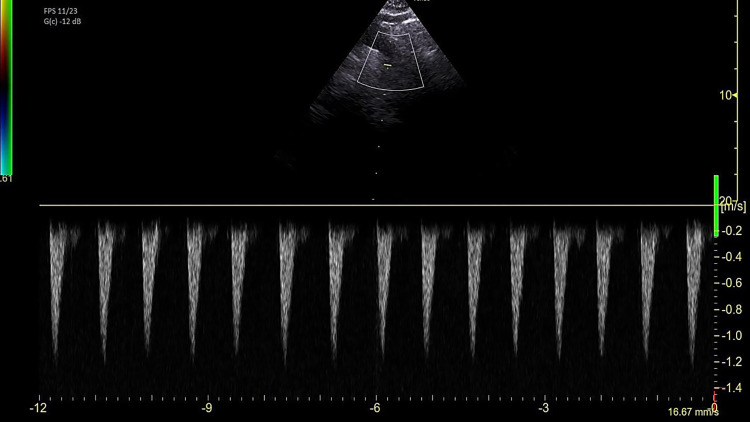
Example of peak velocity of aortic blood flow (V_peak_) sampled at the descending aorta site. Images are taken with the aid of color doppler to recognize blood flow and ensure the best alignment
